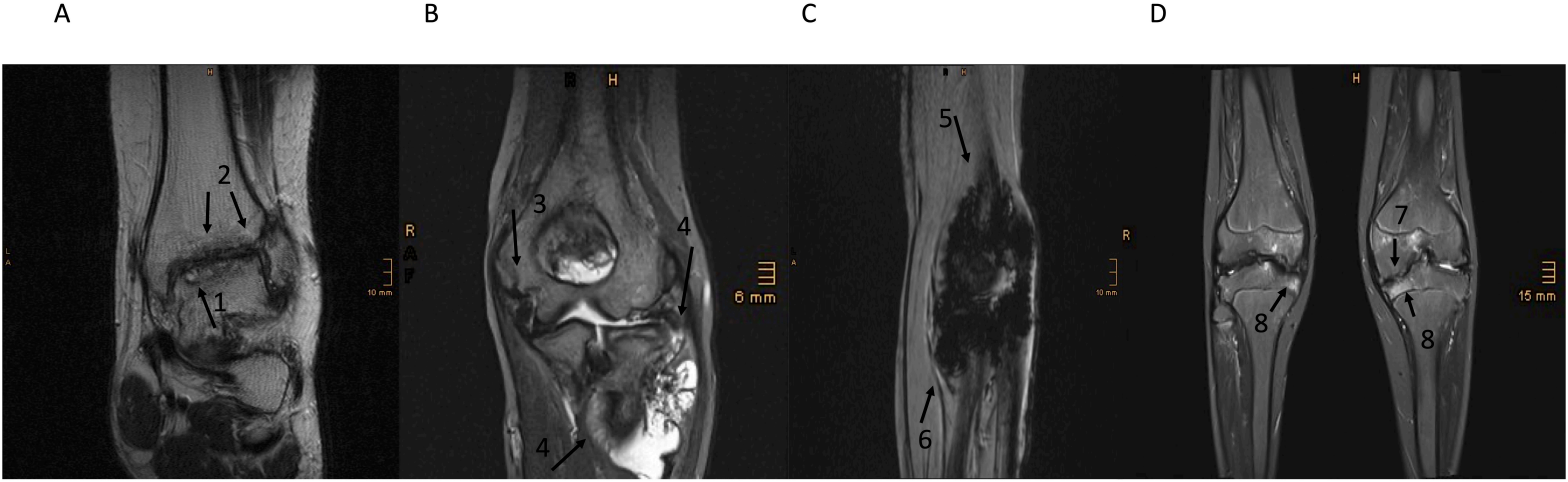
MRIs from patients with an IPSG score ≥ 8. (A) Left ankle pt. ID sevHA15 (coronal T2-weighted turbo spin echo): (1) subchondral cysts and (2) reduced joint space and joint incongruence. (B) Left elbow pt. ID sevHB35 (coronal T2-weighted turbo inversion recovery): (3) osseous joint destruction and (4) mass synovial hypertrophy with hemorrhagic parts. (C) Right elbow pt. ID sevHB35 (sagittal T2-weighted): (5, 6) pronounced hemosiderin artifacts appearing as dark or low-signal-intensity areas. (D) Knees pt. ID sevHB33 (coronal T2-weighted turbo inversion recovery): (7) osteophyts appearing as bony outgrowths, osseous joint destruction and joint incongruence, (8) bone marrow edema showing high signal on T2 weighted images.
